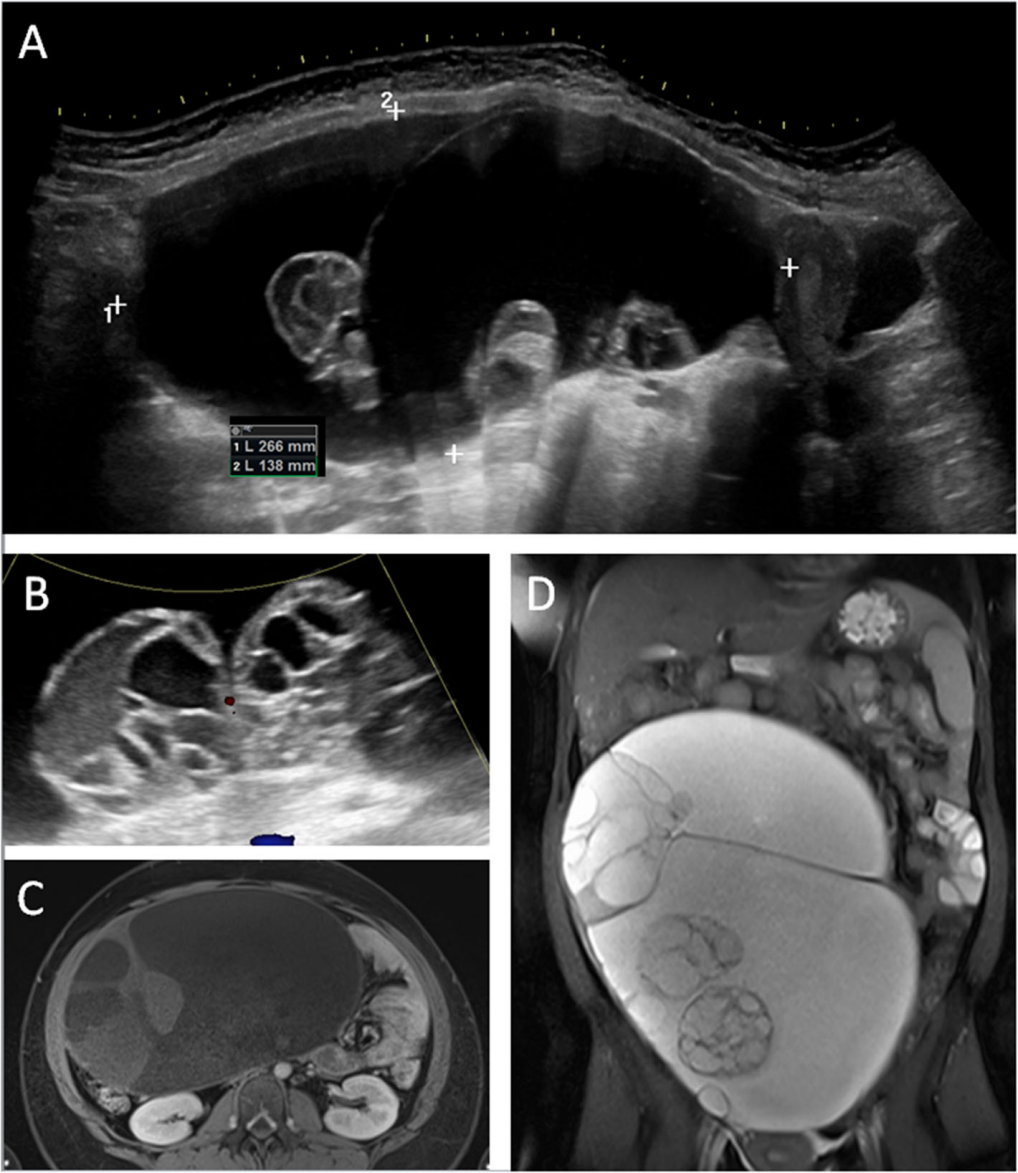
a Abdominal ultrasound (panorama mode in sagittal plane) revealing the large cystic mass filling nearly the whole abdomen with a cranio-caudal diameter of approximately 27 cm. The main cystic tumor consists of many smaller cyst-in-cyst structures. b Ultrasound image with the typical cyst-in-cyst sign suspicious for diagnosis of hydatid disease in stage CE2. c, d Magnetic resonance imaging scan of the abdomen (b, T1 sequence axial; c, T2 sequence coronal) showing the marked-off large cystic mass filling nearly the whole abdomen and displacing parenchymal organs and intestinal structures
